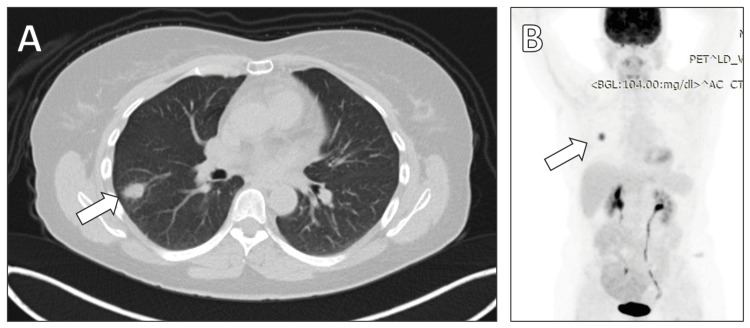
Preoperative diagnostic imaging (A) A computed tomography (CT) scan of the chest showing a neoplastic mass in the right lower lobe of the lung. (B) Positron emission tomography/CT (PET/CT) imaging of the lung neoplasm with a maximum standardized uptake value (SUVmax) of 8.2, indicating high metabolic activity.
